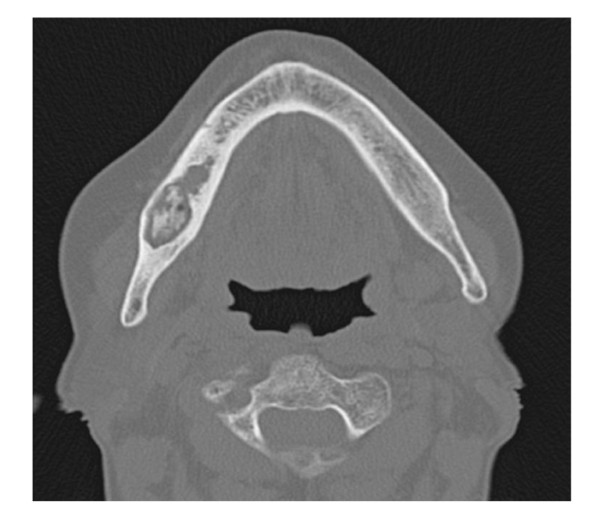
Clinical Onset: computed tomography scan shows bone destruction.
